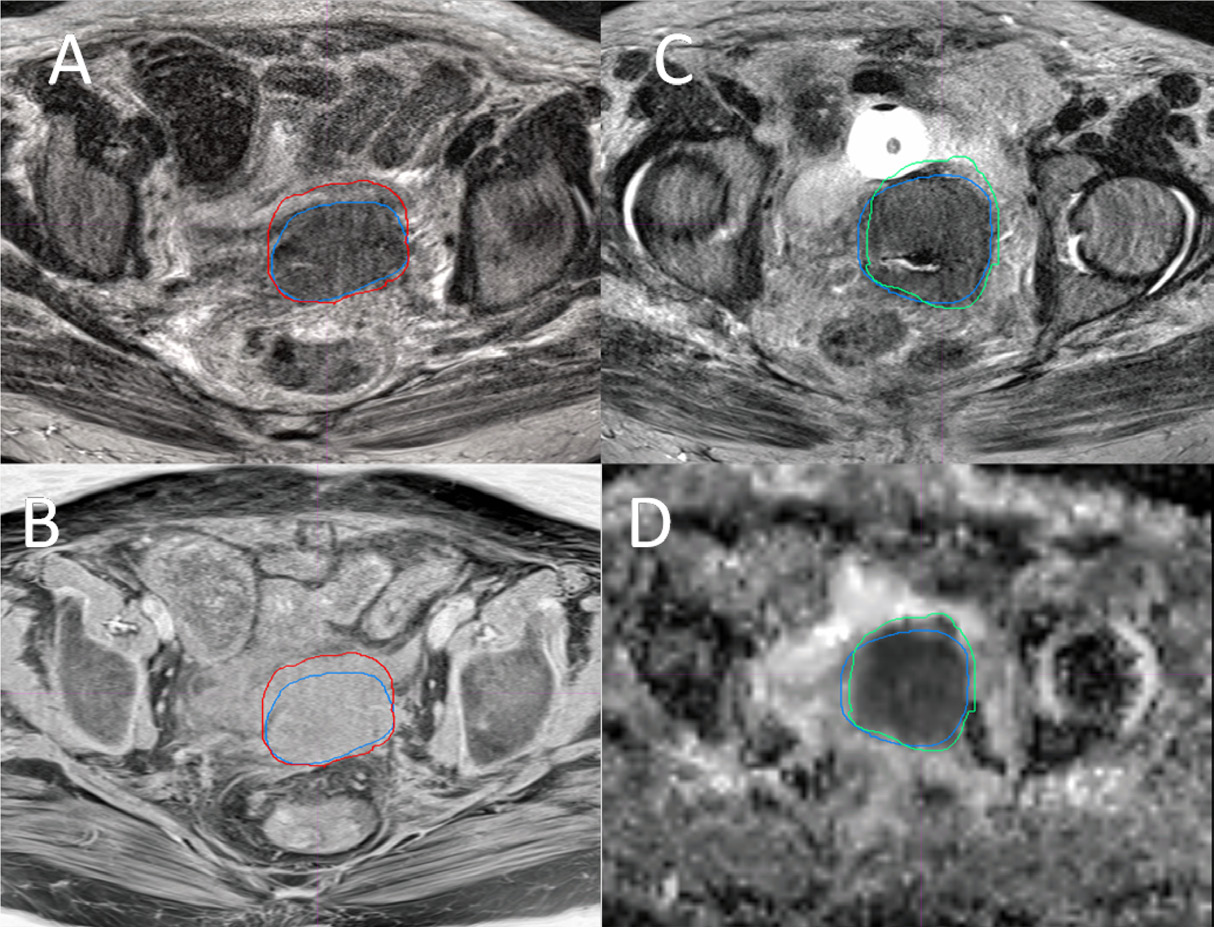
Overlay of the GTVs from four MRI sequences of a Stage IVA cervical tumor Overlay of GTV-T2 (blue) and GTV-T1 (red) on a T2 (A) and a T1 (B) slices, and overlay of GTV-T2 (blue) and GTV-ADC (green) on a T2 (C) and an ADC (D) slices, where GTV-T2 = 98.4 cm^3^, GTV-T1= 110.2 cm^3^, and GTV-ADC = 90.8 cm^3^.
